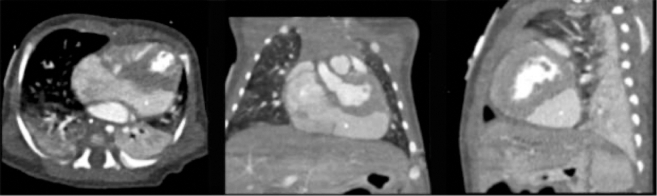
Postnatal Cardiac Computed Tomography Angiography Depicting Enlarged Coronary Sinus (Asterisk) Along the Left Posterior Atrioventricular Groove on Axial, Coronal, and Sagittal Images
